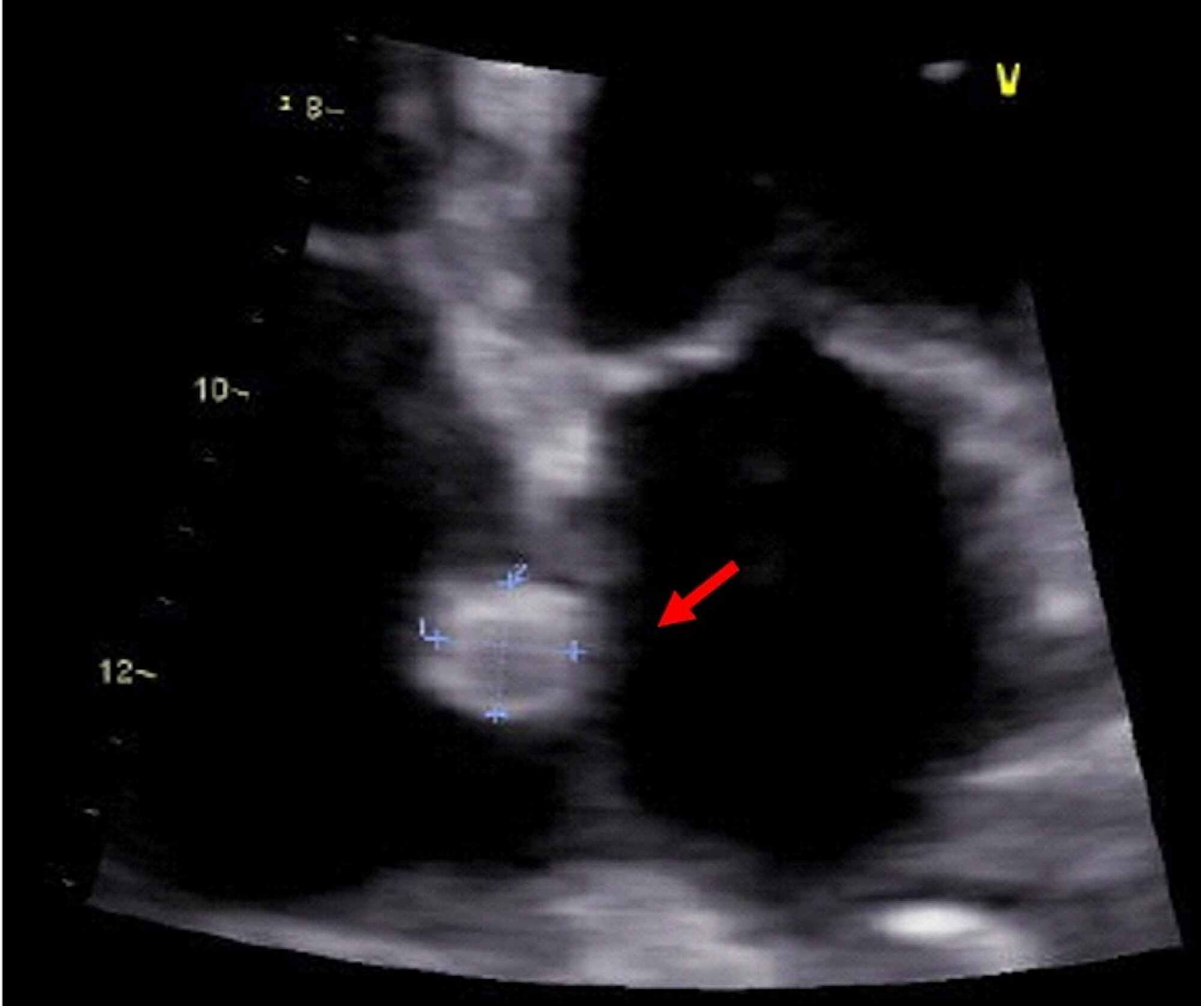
Echocardiogram showing a nodular image adherent to the right atria (arrow).
